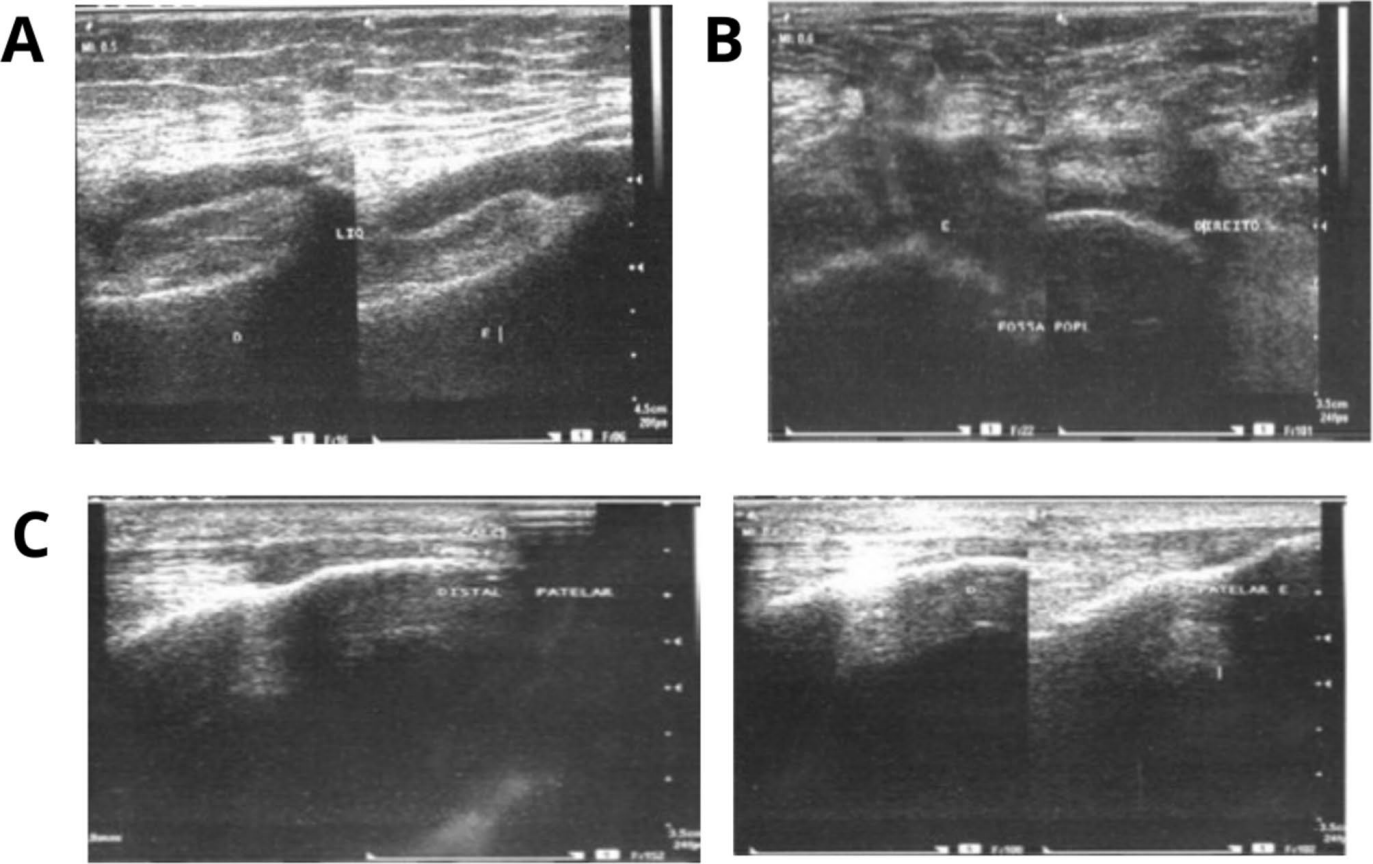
Knee ultrasonography. (A) Joint effusion. (B) Popliteal cyst. (C) Patellar tendinitis.
